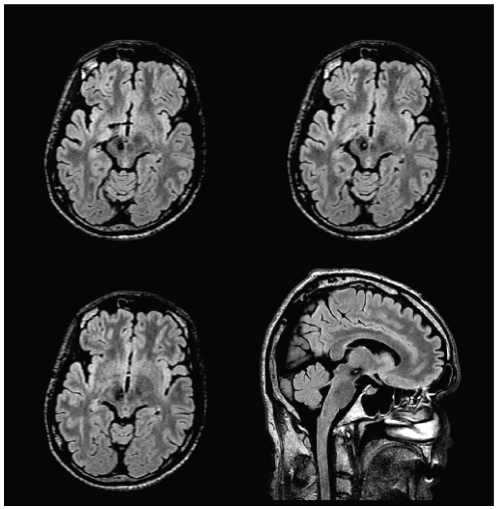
Preoperative FLAIR images show the right perirubral mesencephalic traumatic haemorrhage. Images are presented in radiological convention.
